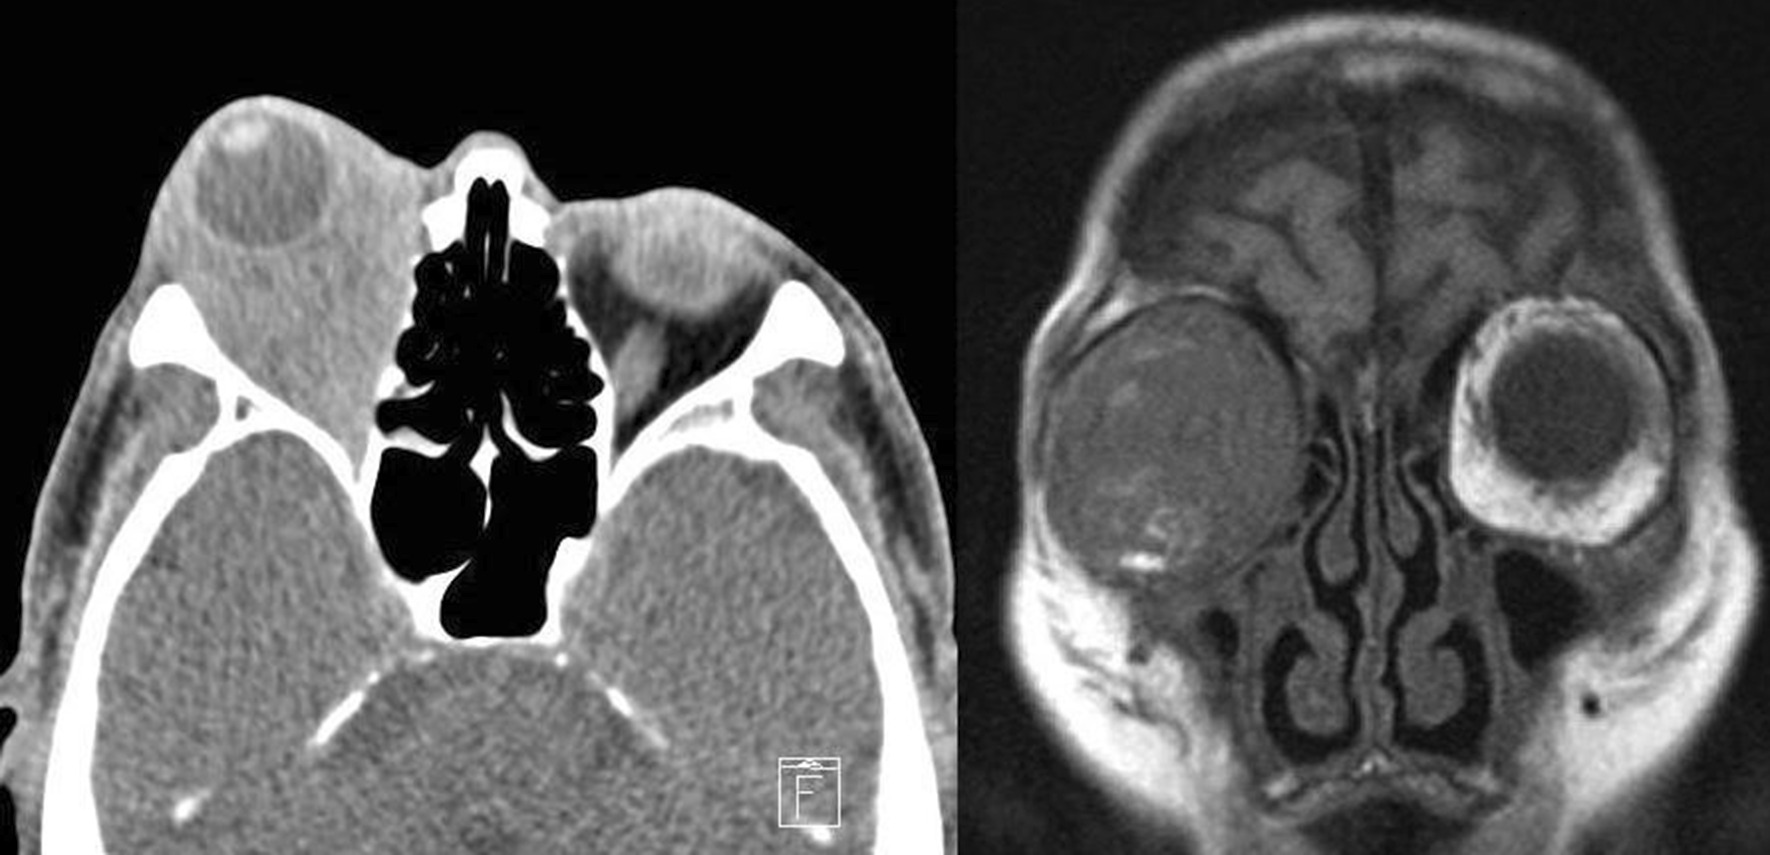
a (left) Computed tomography examination of the right orbit showing a soft tissue shadow that occupies the roof of the right eye with no bone abnormality or damage. No abnormalities were noted in the brain parenchyma within the imaging range. b (right) Magnetic resonance imaging suggested an intraocular tumor probably because of an inflammatory pseudotumor
